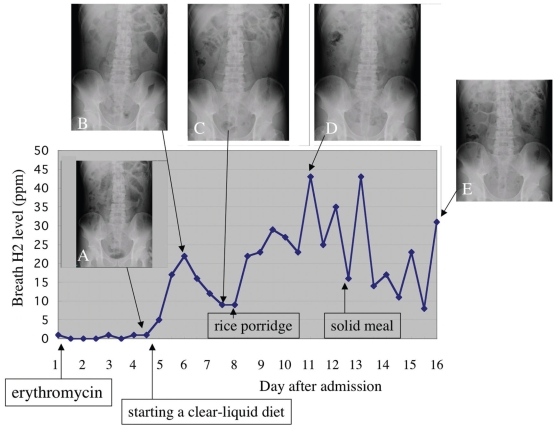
Clinical course of case 2 after a treatment with erythromycin. Changes in breath hydrogen concentration and abdominal plain radiographs on representative dates are demonstrated.
